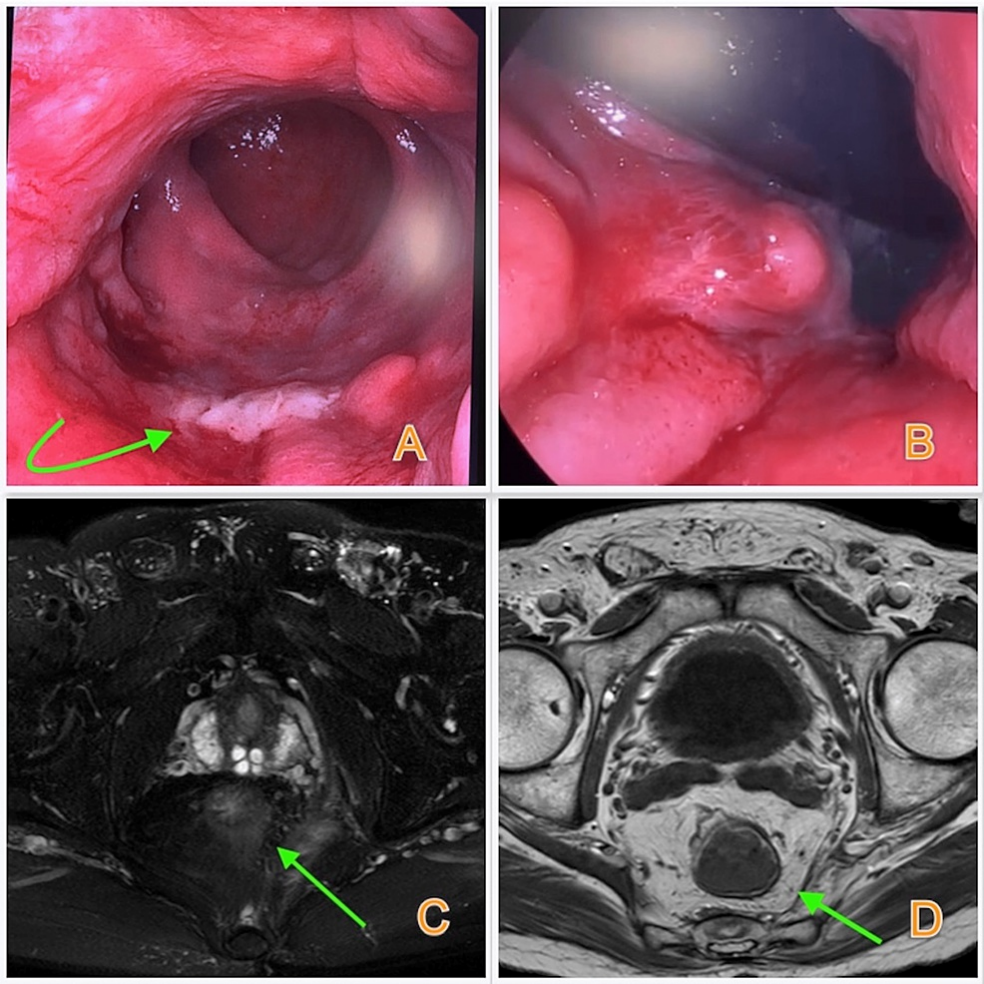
View of colonoscopy and MRI after EVT A) Control colonoscopy revealing the perforation side totally closed with a granulation tissue (marked with green arrow); B) Retrovert view of the granulation tissue; C-D) MRI that confirms total resolution of the abscess (marked with green arrows) MRI: Magnetic resonance imaging; EVT: Endoluminal vacuum therapy
